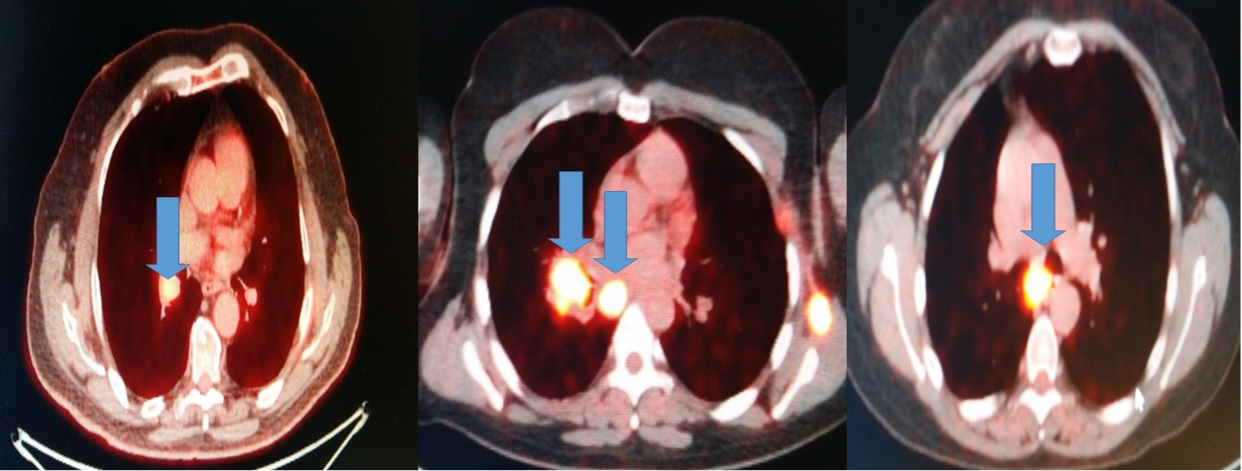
Blue arrows indicate the positive lymphnodes that were punctured (SUV>3).
